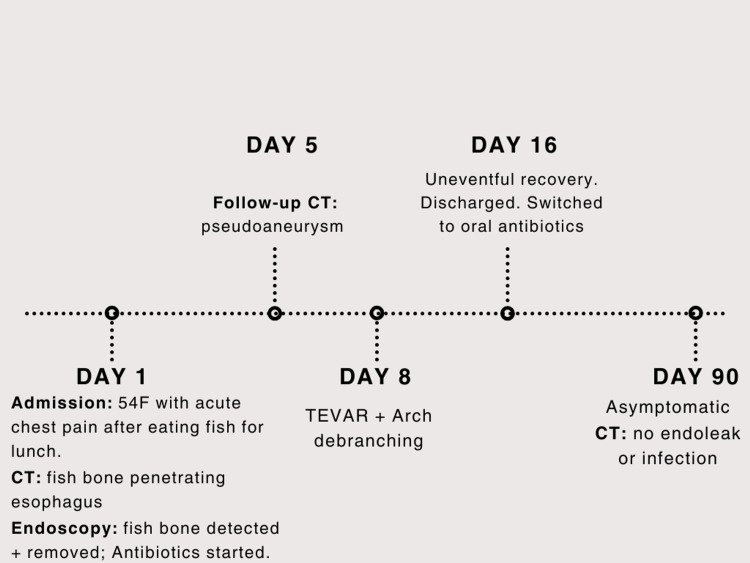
Important milestones during patient management CT: computed tomography; TEVAR: thoracic endovascular aortic repair
